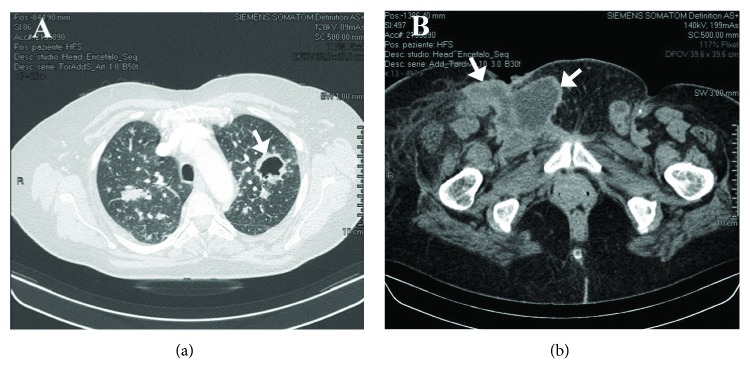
Further systemic tumor progression during the second-line chemotherapy. CT scan showing multiple lesions in the lungs (a), some excavated (arrow), and matted metastatic lymph nodes in the iliac/inguinal region (b) (arrows).
